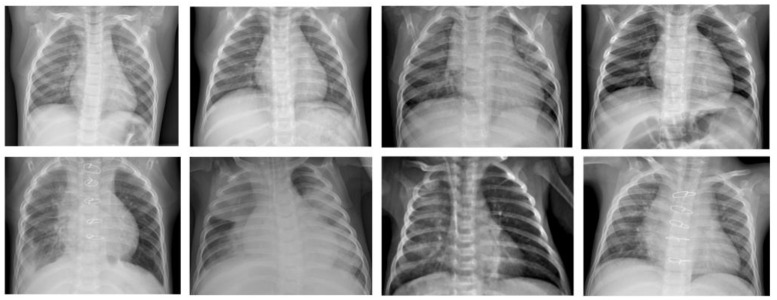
Chest X-ray samples used in this paper [24]. Top row: X-ray samples with no pneumonia. Bottom row: X-ray samples with pneumonia.
